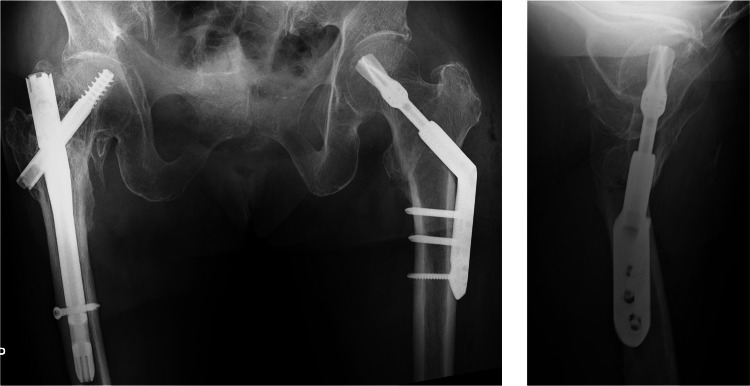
Postoperative X-ray images of the hip. Internal fixation by DHS was performed.
